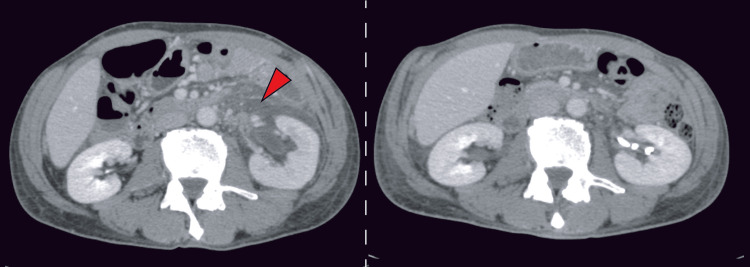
Contrast-enhanced abdominal CT after treatment Contrast-enhanced abdominal CT on hospital day two (left panel) and one month after ureteral stent placement (right panel). The perirenal fluid collection around the left kidney (red arrow) and hydronephrosis observed on hospital day two showed marked improvement after stent placement.
